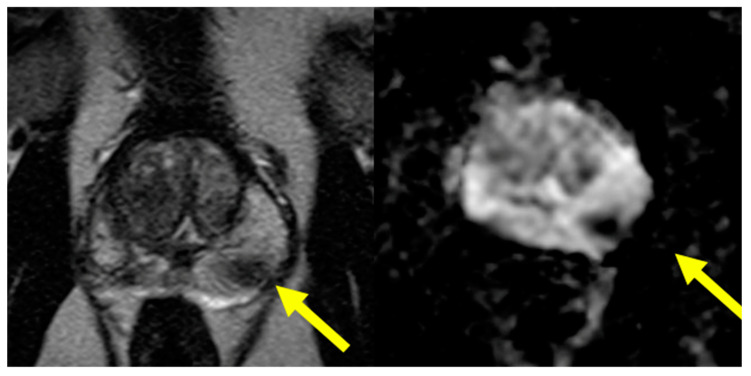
Axial T2-weighted (left) and axial ADC images (right) with arrow identifying the ROI.
